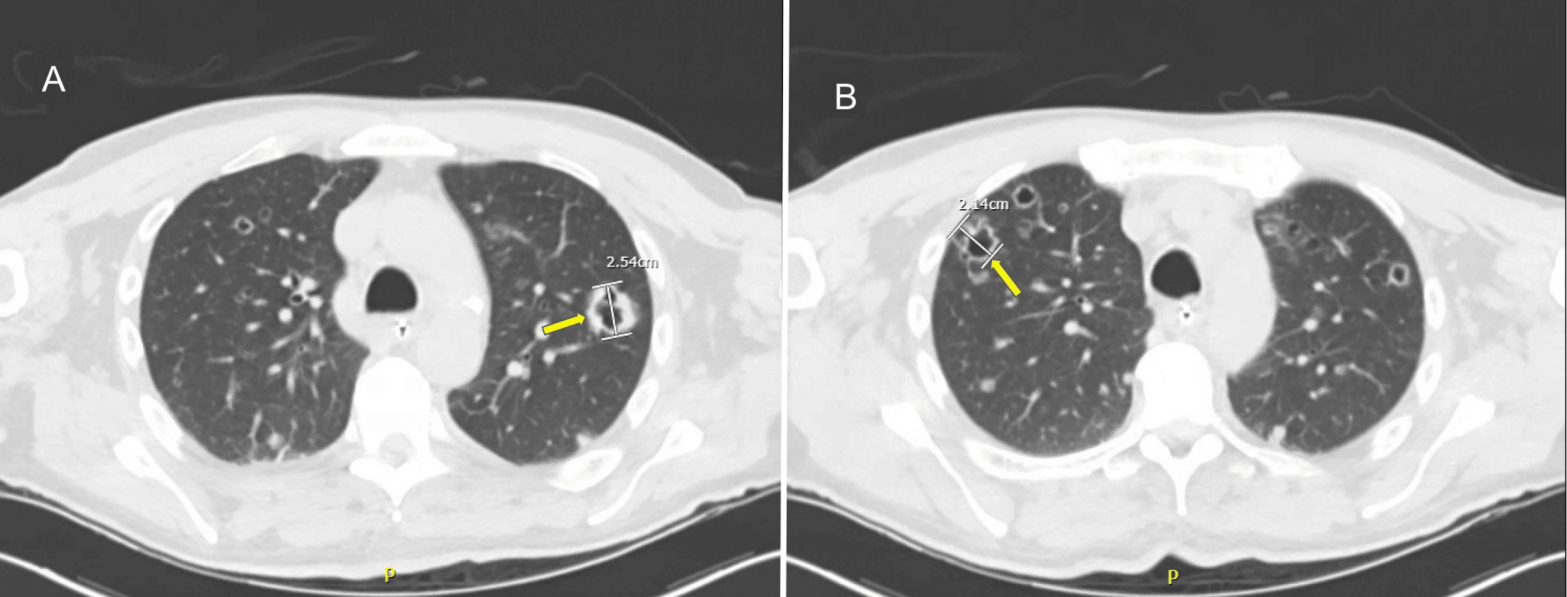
CT scan of chest revealing multifocal cavitary lesions within left (A) and right (B) lobes secondary to septic pulmonary embolization. Yellow Arrows: Cavitary lesions
